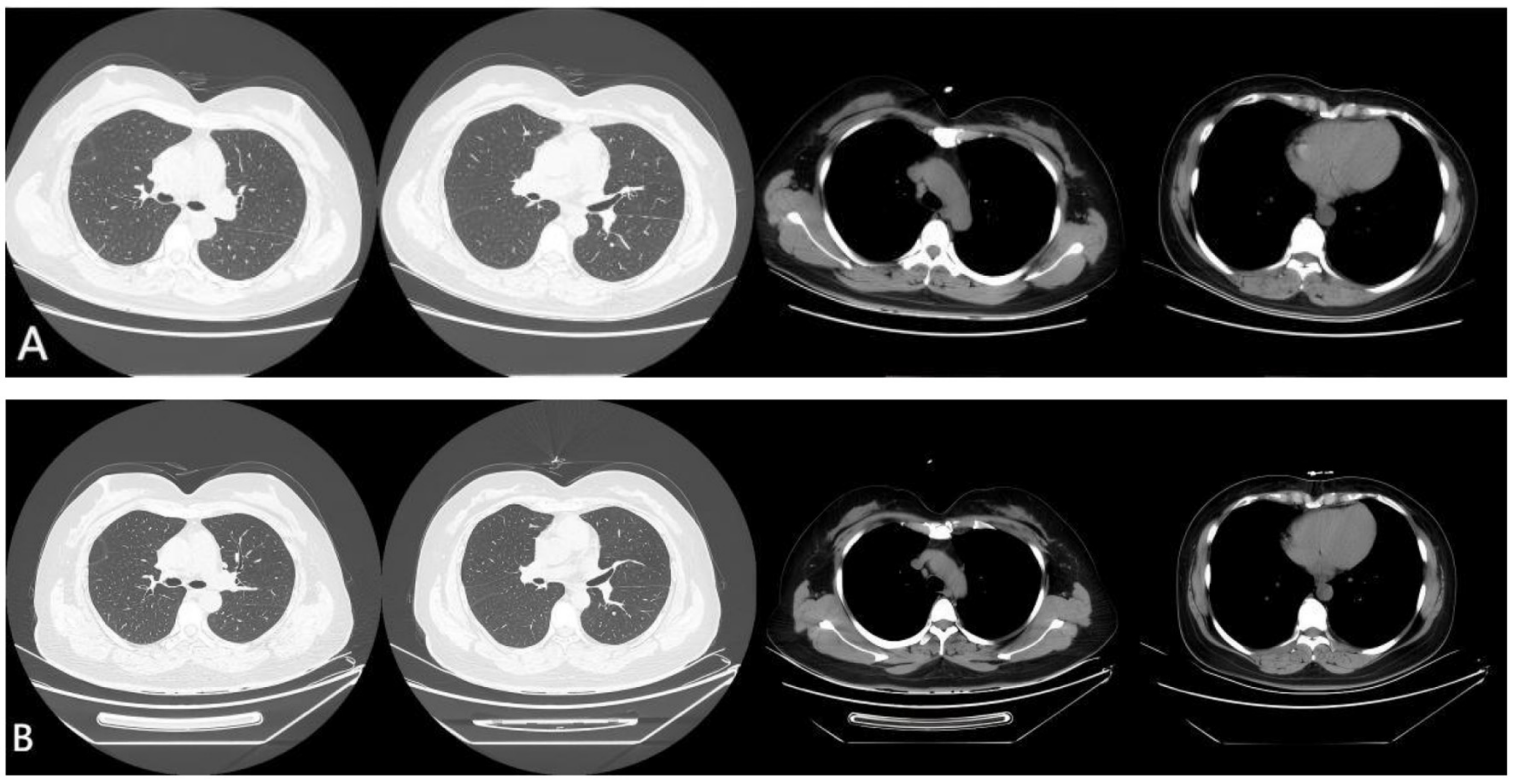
Follow-up chest CT showed a complete withdrawal (A) and no recurrence of the pulmonary nodules, subpleural nodules and bilateral pleural effusion (B).
